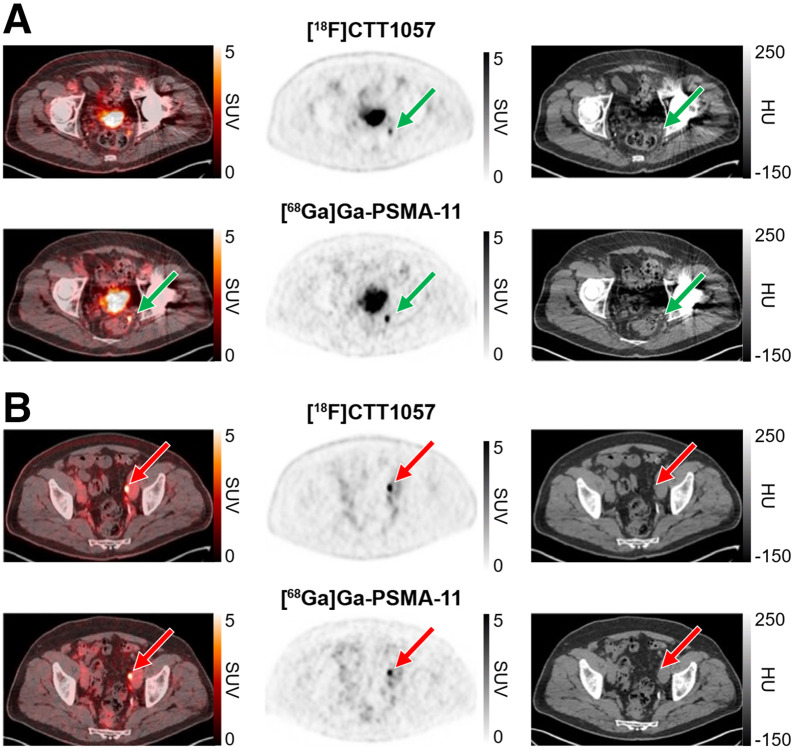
[^18^F]CTT1057 and [^68^Ga]Ga-PSMA-11 PET/CT scan images (axial slices) showing true-positive lesions in pelvic lymph node metastases. True-positive [^18^F]CTT1057 and [^68^Ga]Ga-PSMA-11 perirectal lymph node (A, green arrow) and left external iliac lymph node (B, red arrows) lesions in 71-y-old patient with BCR (pT3a; Gleason score, 7 [3 + 4]) diagnosed 67 mo after initial RP (PSA level at time of PET scans, 0.25 ng/mL). HU = Hounsfield unit.
